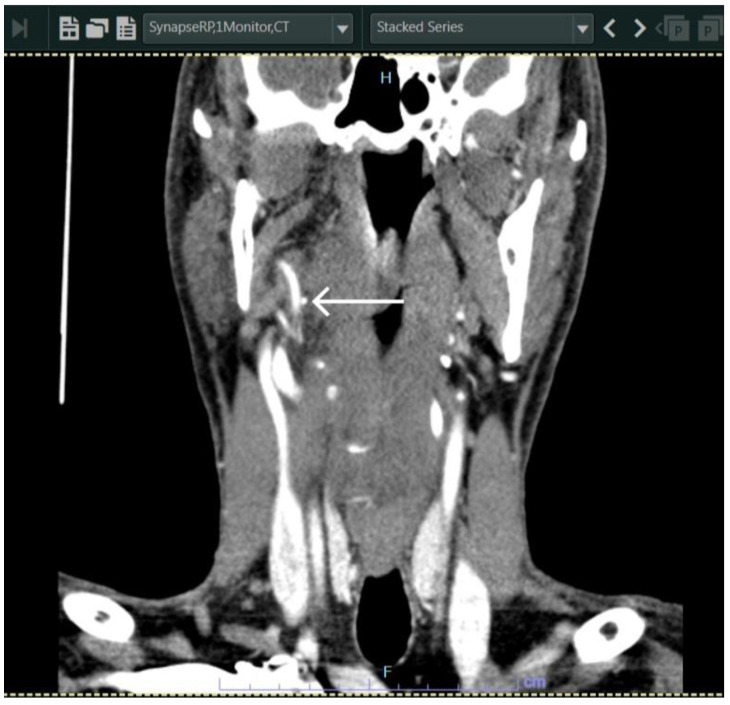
Coronal section of CT with contrast taken immediately preoperatively, showing the fractured needle in close proximity (arrow) to a large vessel. The needle was identified in the most medial part of the right medial pterygoid muscle, close to the plane between the medial pterygoid muscle and the superior pharyngeal constrictor muscle. Consent was obtained from the patient to operate under general anesthesia using either a transoral or transcervical approach. The patient was placed in the supine position, general anesthesia was induced, and the oral cavity was prepared and draped in. Oral intubation was used and the endotracheal tube was inserted and placed on the other side (left side of the oral cavity) so as not to interfere with the field of interest.
